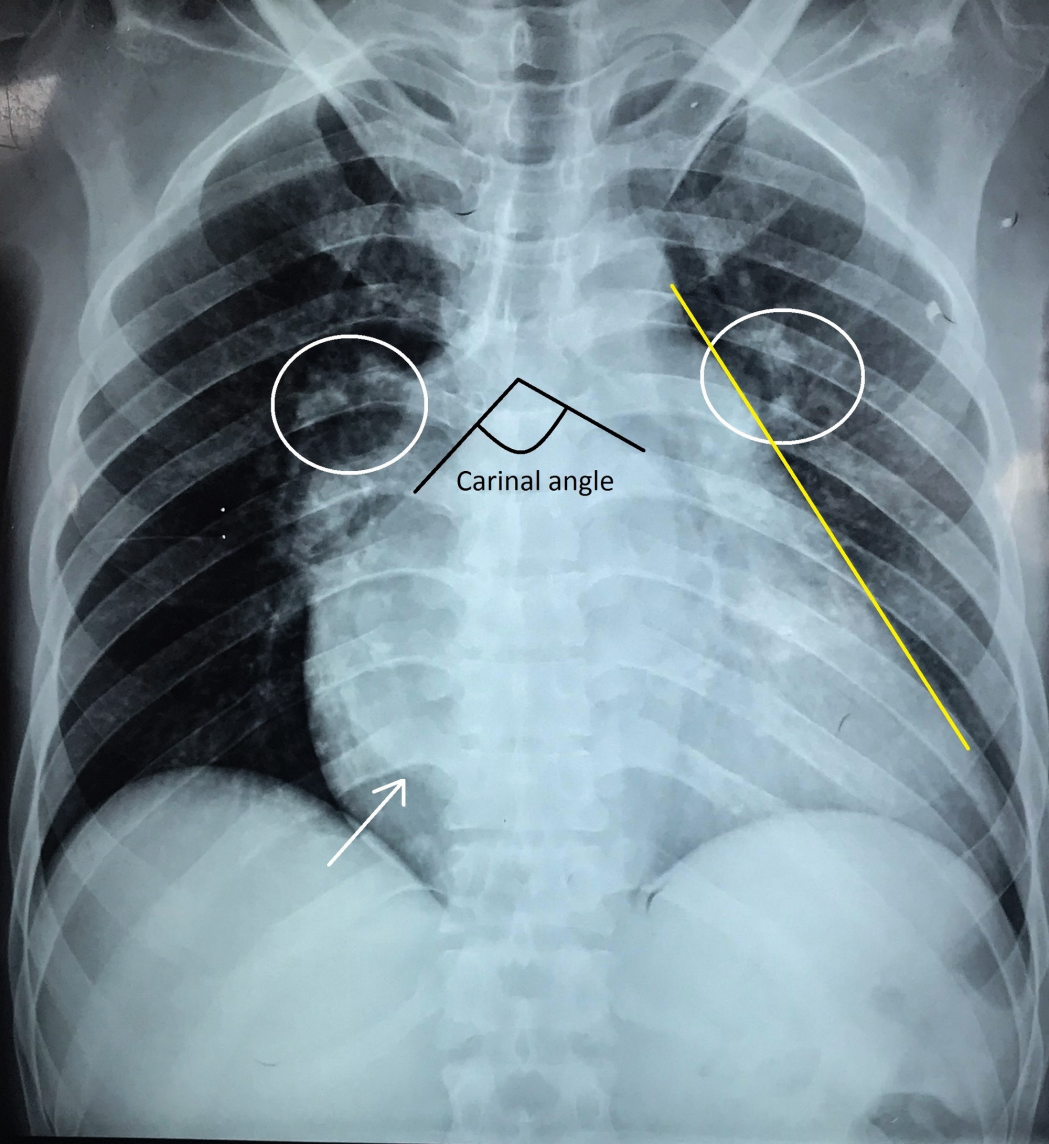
Chest radiograph (posteroanterior view) showing cardiomegaly with cardiothoracic ratio 70%, straightening of left heart border (yellow line), double right heart border (white arrow), cephalization (white circle), and widened carinal angle typical of left atrial enlargement The cardiac border was displaced laterally and downwards implying left ventricular enlargement.
